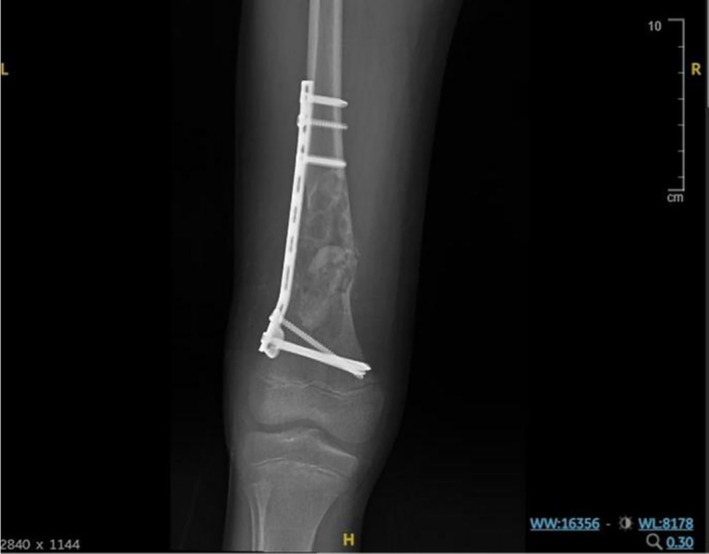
Postoperative anteroposterior radiograph showing intact distal femoral fixation with preserved physis and knee joint space. Plate‐and‐screw fixation remains in satisfactory position with maintained alignment and no evidence of implant failure. The distal femoral epiphysis and physis are intact, and the knee joint space is preserved without radiographic signs of effusion.
